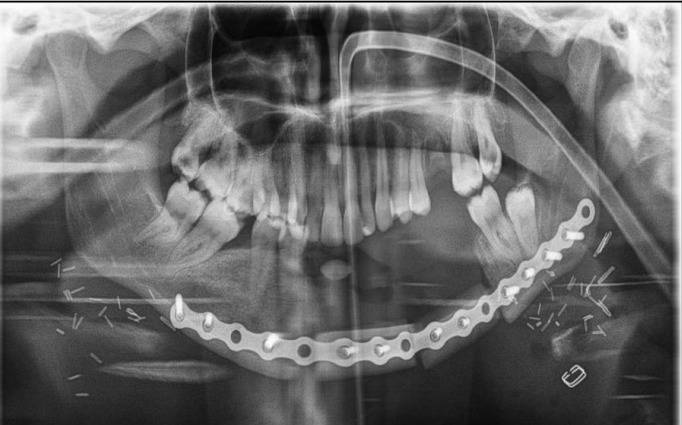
Post-op panoramic x-ray of jaw reconstruction.
